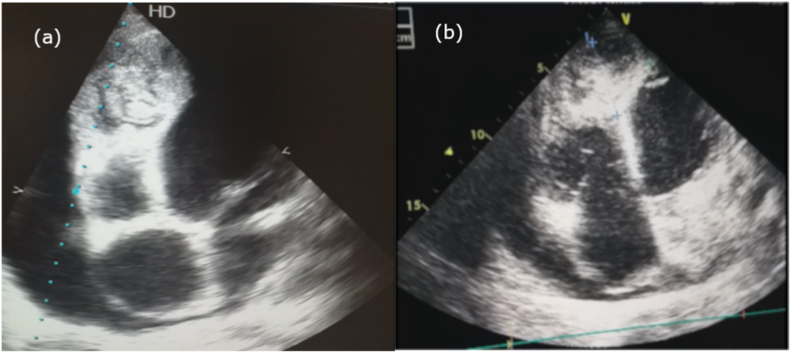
Transthoracic echocardiography shows (a) a hydatid cyst in the distal part of the interventricular septum and apex of left ventricle (b) dimensions of the cyst (6 × 4.3 cm).
